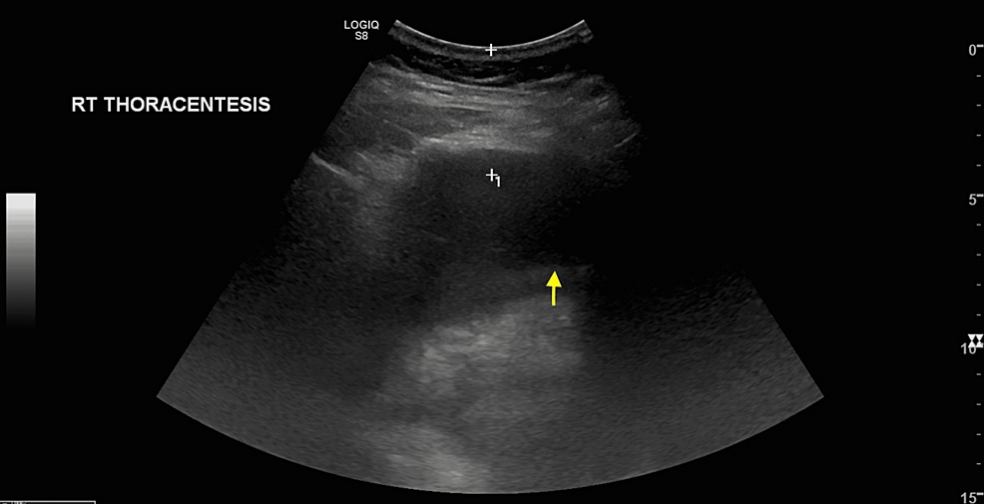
Ultrasound showing a moderate-sized pleural fluid pocket (yellow arrow).
